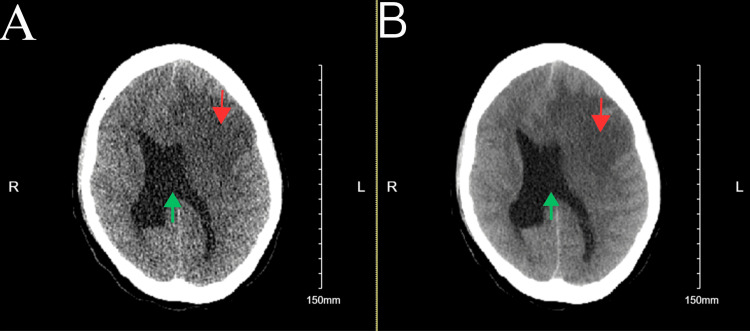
Computed tomography (CT) scan of the head without contrast demonstrated a large heterogeneous mass centered about the left frontal lobe with stable locoregional mass effect findings (red arrows); specifically persistent left-to-right midline shift of 9 millimeters (green arrows) with subfalcine and left uncal herniation. (A) Computed tomography scan without contrast exhibiting a more uniform grayscale and less differentiation between different tissue densities. (B) Computed tomography scan without contrast exhibiting more defined parenchymal structures and a greater differentiation between tissues. Red arrows: Demonstration of the large heterogeneous mass centered about the left frontal lobe with stable locoregional mass effect findings. Green arrows: Persistent left-to-right midline shift of 9 millimeters.
